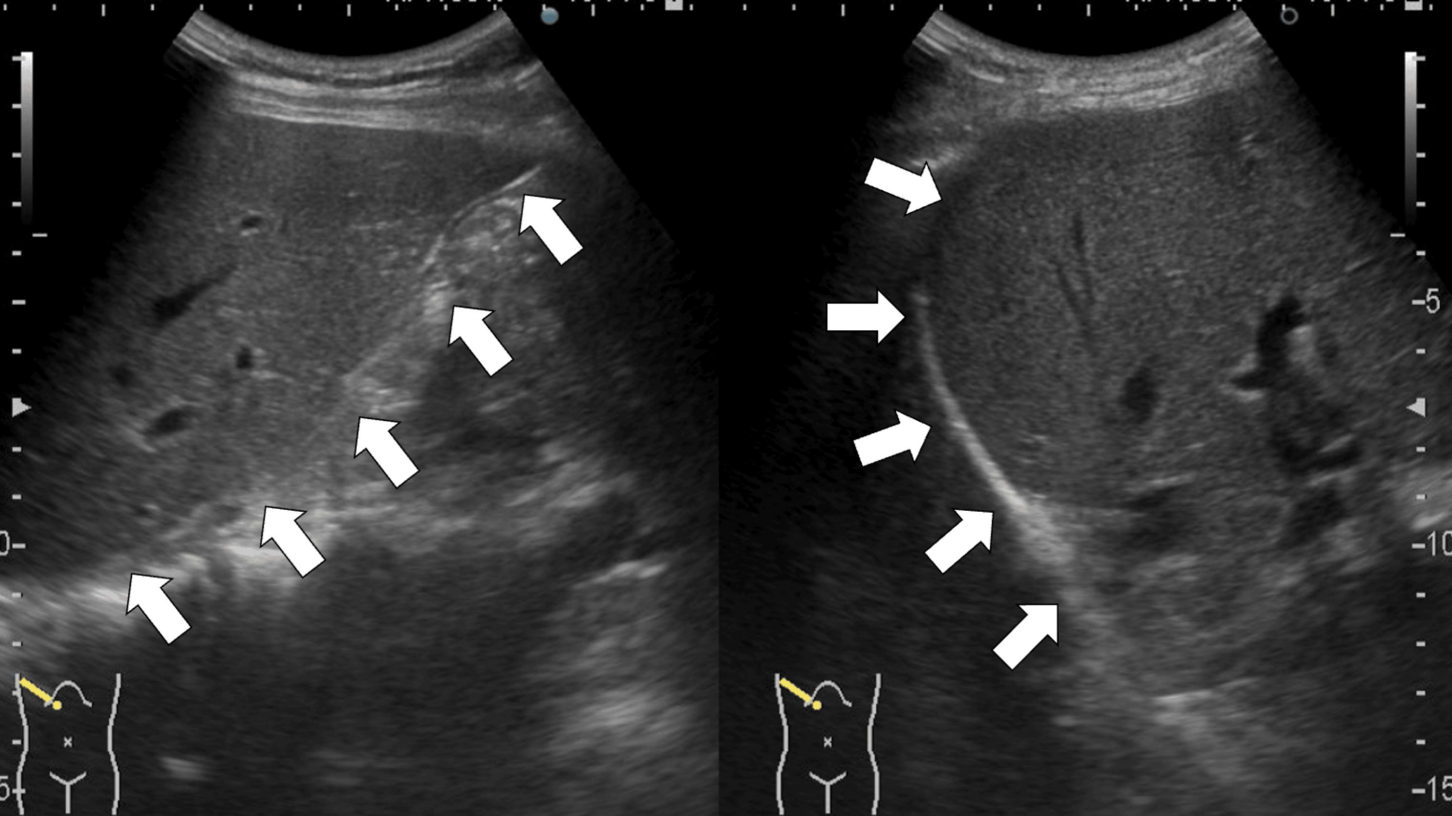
Abdominal ultrasound revealing no hepatomegaly (white arrows)
